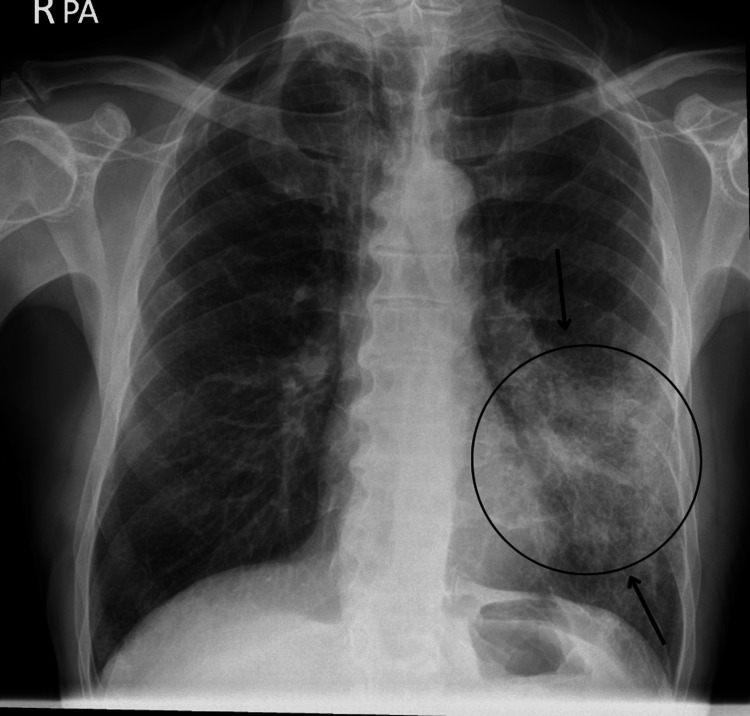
Chest X-ray at presentation showing left lower zone opacification (arrow+circle) consistent with pneumonia Black arrows and the circle indicate areas of consolidation in the left mid and lower lung zones, consistent with community-acquired pneumonia. No evidence of pleural effusion or pneumothorax is present.
